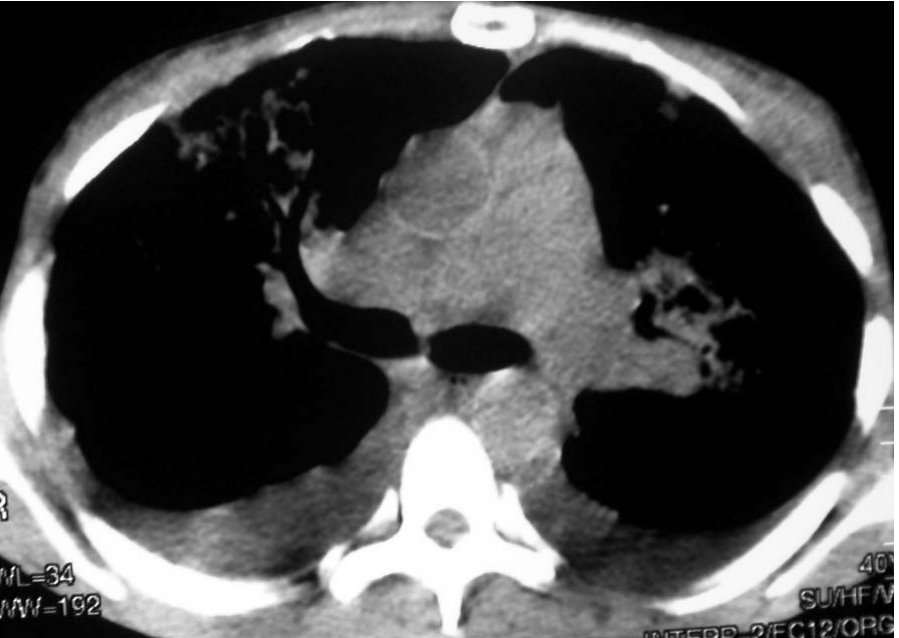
CT scan (mediastinal window) at the level of the carina of a patient with pulmonary KS shows bilateral pleural effusion.
